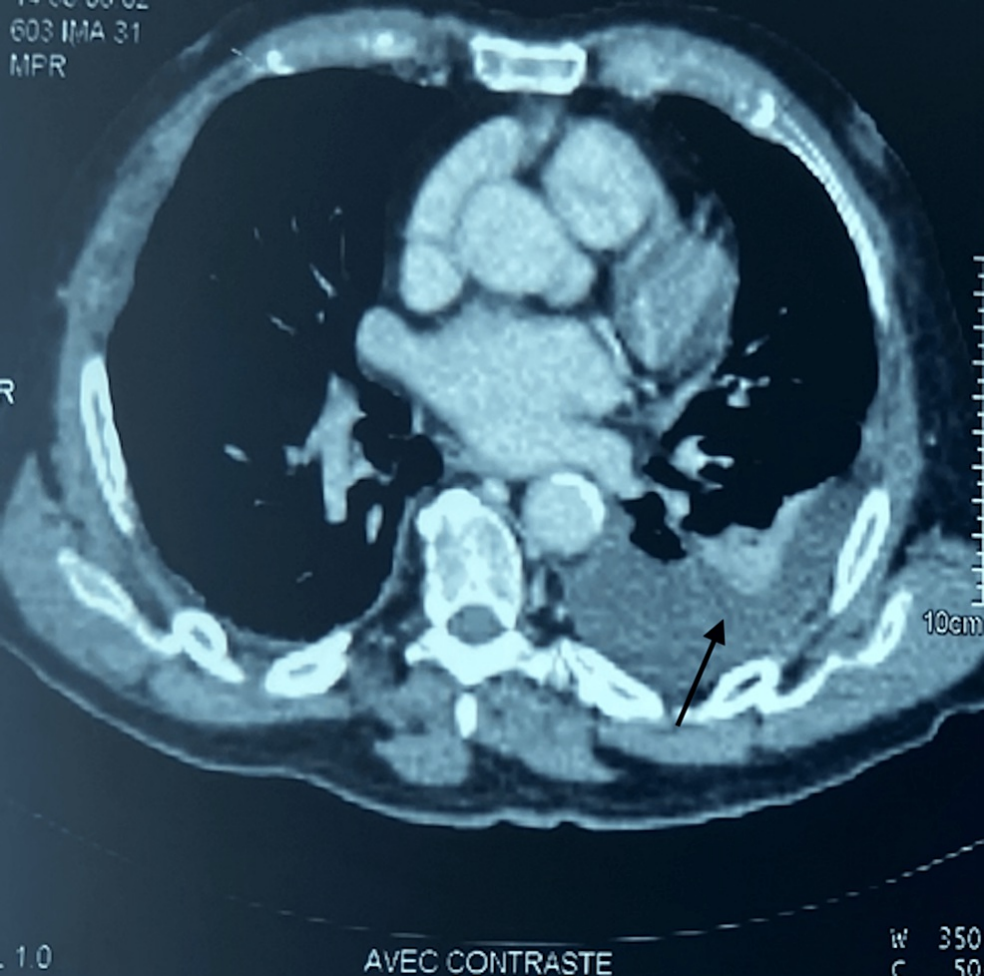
The chest CT scan revealed a collapsed lung along with a moderate-sized pleural fluid effusion (arrow) on the left side
